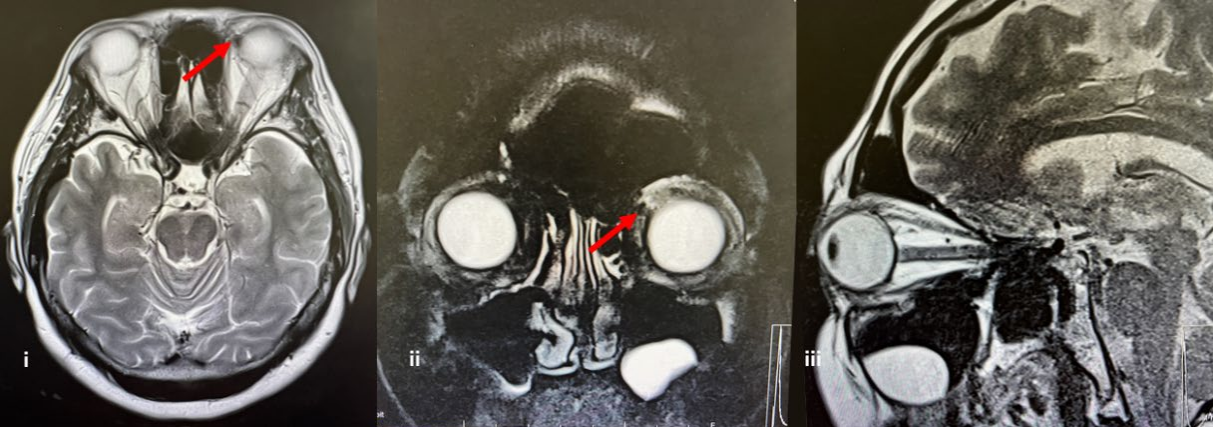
(i) Axial MRI demonstrates bilateral eye globes, ‘with f focal thinned lesion at the left supetior mcVial orbit of the lefc eye, mrasuring 0.8 (AP) × 0.9 (wt)× 1.9 (Ht) cm. Tie lesion exhibited a low signal on T1-weighted imaging, a high signal on T1 fat-suppressed imaging and minimal enhancement, consistent with a cavernous haemangioma. (ii) Coronal view, (iii) Sagittal view.
